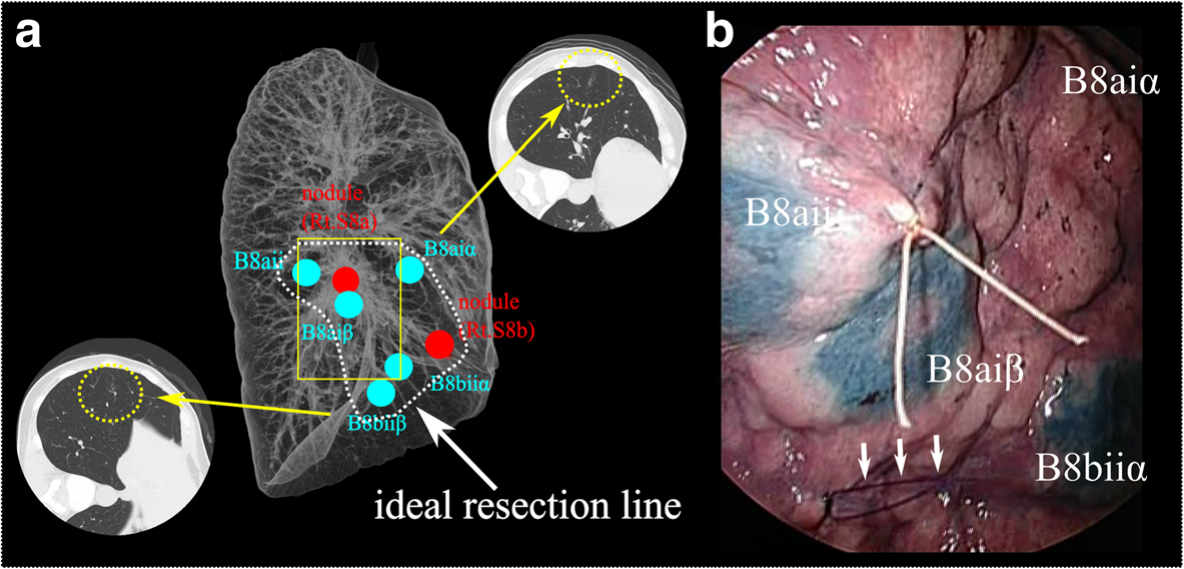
a Three-dimensional configuration of the CT for “mapping” for the first operation. b A thoracoscopic view corresponding to the yellow square in a. The “standing stitch” with blue suture (white arrows) near a marking spot indicates the resection line between S8 and S9 and a silk stitch just above the nodule in right S8a. The geometric information of ideal resection line was obtained from marking spots and blue sutures were placed along the resection lines
